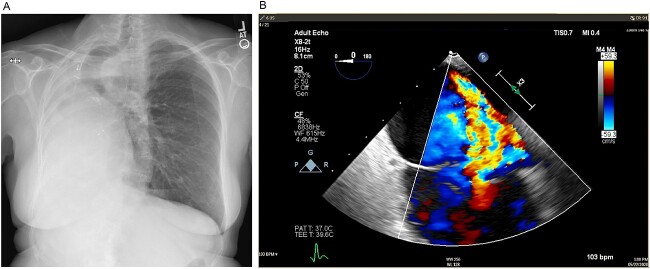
(A) Preoperative posteroanterior view chest X-ray showing left to right mediastinal contents shifted and a clear left lung. (B) Preoperative transesophageal echocardiogram with doppler showing severe tricuspid regurgitation with no coaptation between the anterior and septal leaflets.
